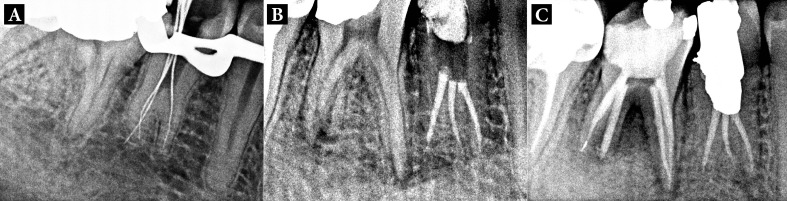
Periapical radiographs of treatment procedures. A: Working length determination. B: Final obturation. C: Healing after 1-year follow-up. Note that during this time, RCT of mandibular right first molar was performed
